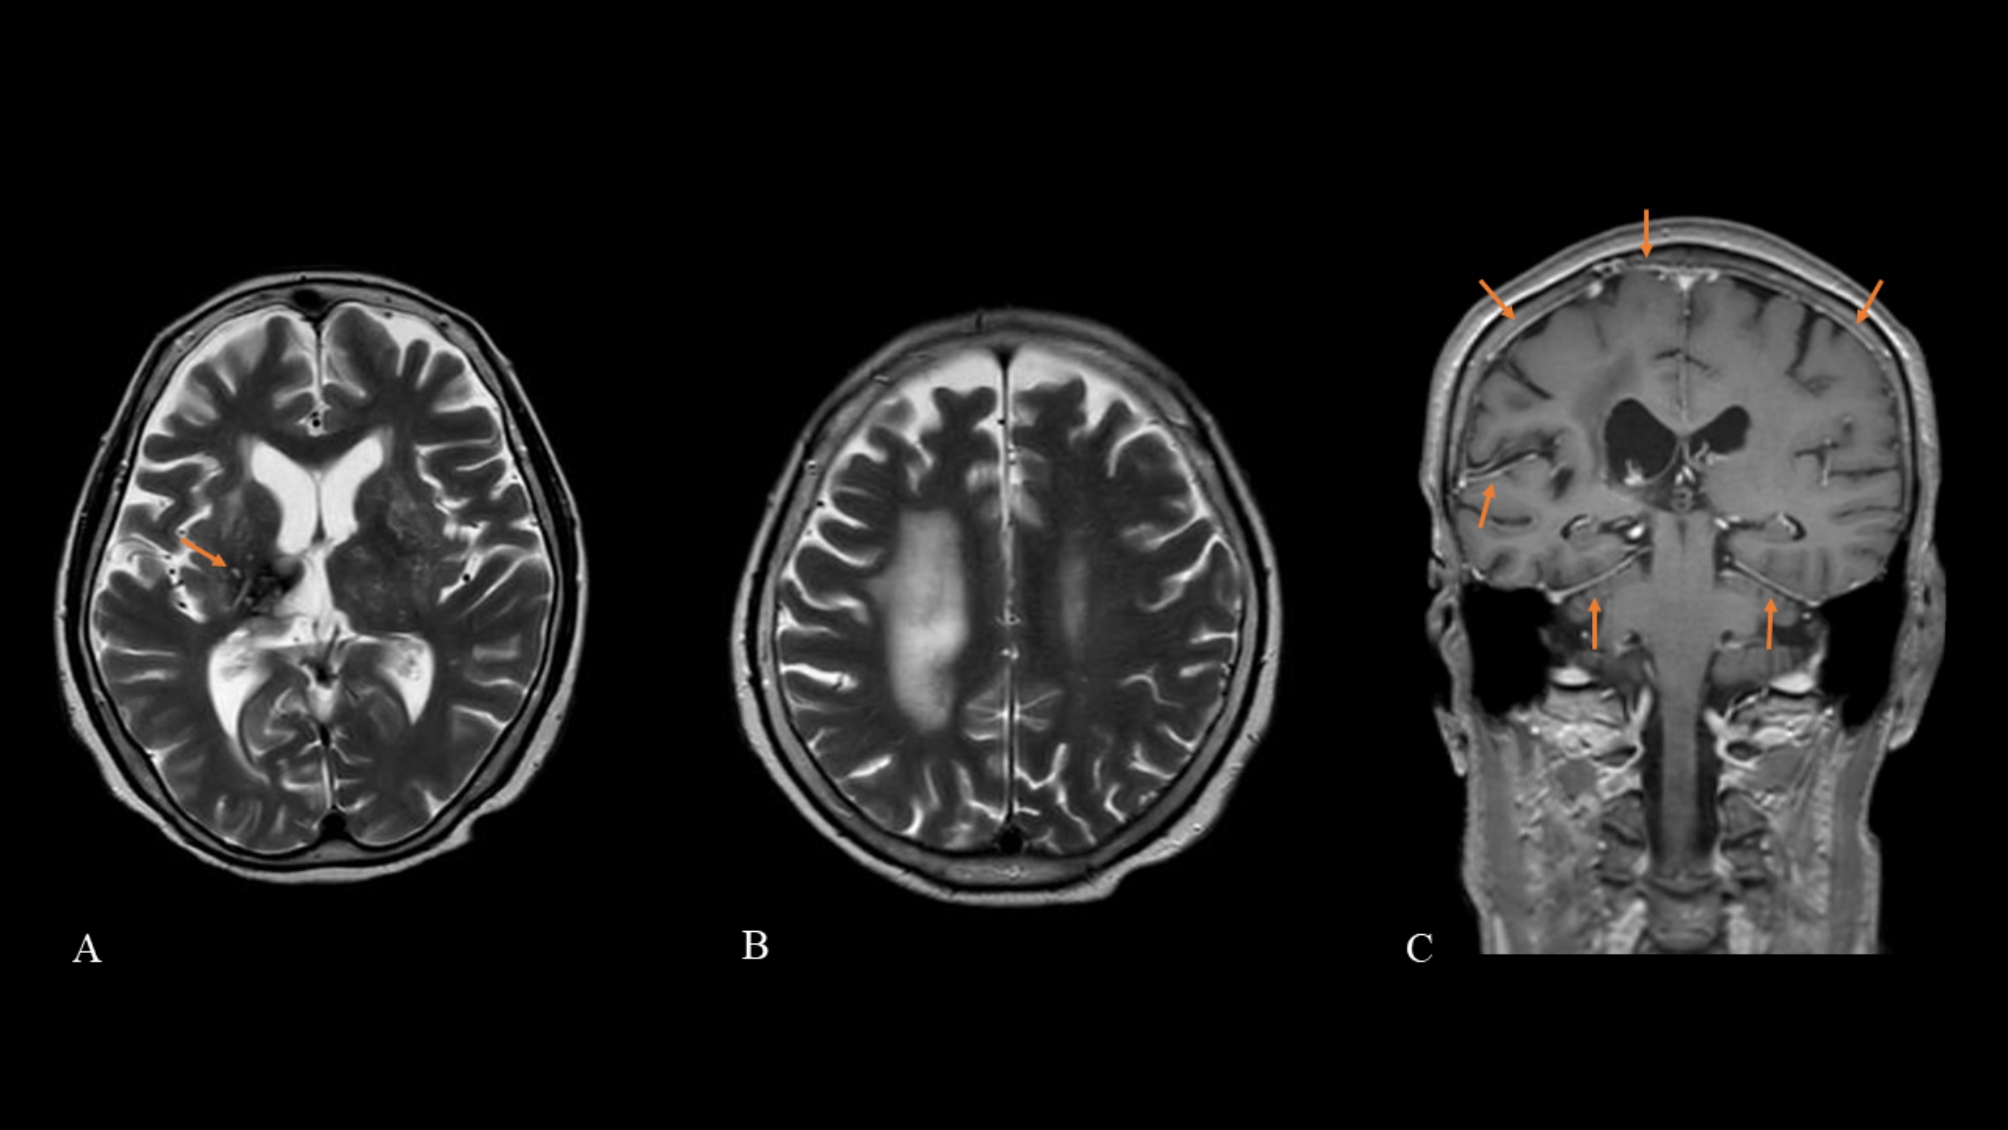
Brain magnetic resonance imaging Brain magnetic resonance T2-weighted images show abnormal signal changes caused by a past right thalamic hemorrhage (arrow) (A) and extended white matter hyperintensity lesions in the deep and periventricular white matter in the right frontal and parietal lobes (B). Gadolinium contrast-enhanced brain MRI scans show heterogeneous thickening of the dura mater (arrows) (C)
